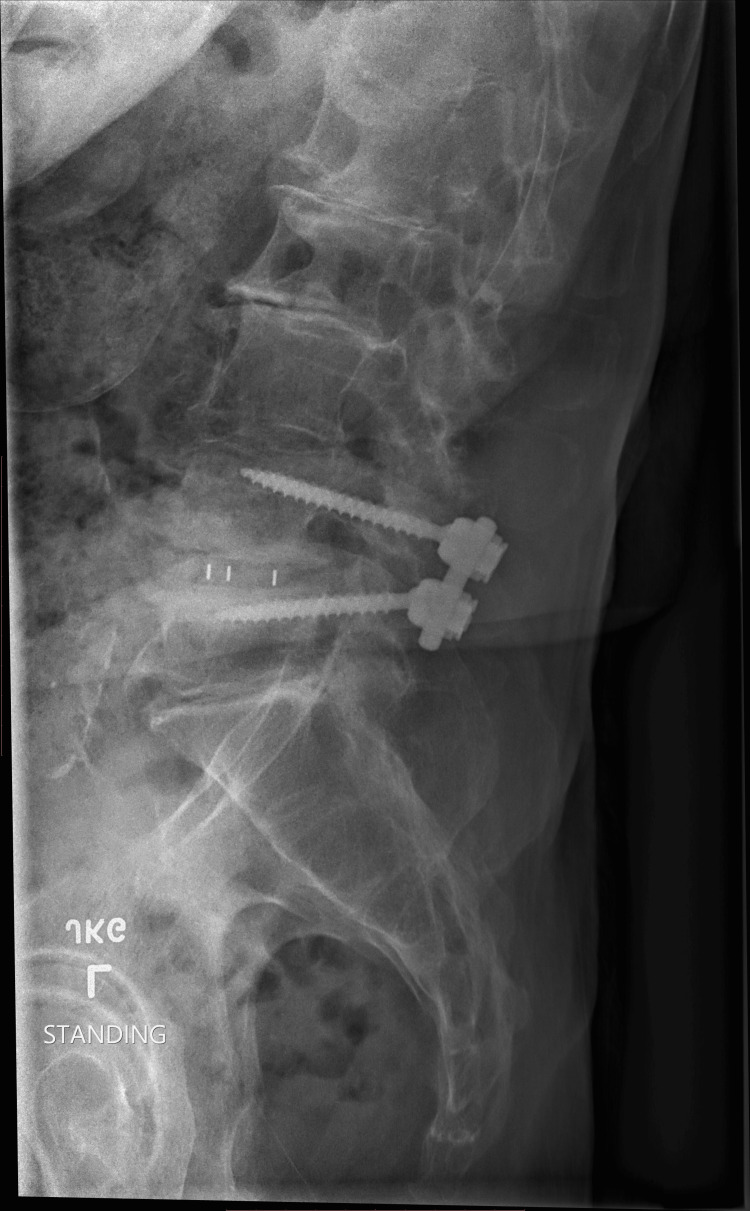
Post-spinal fusion surgery This radiograph shows spinal stenosis titanium hardware that was installed at the L4-L5 level during surgery.
